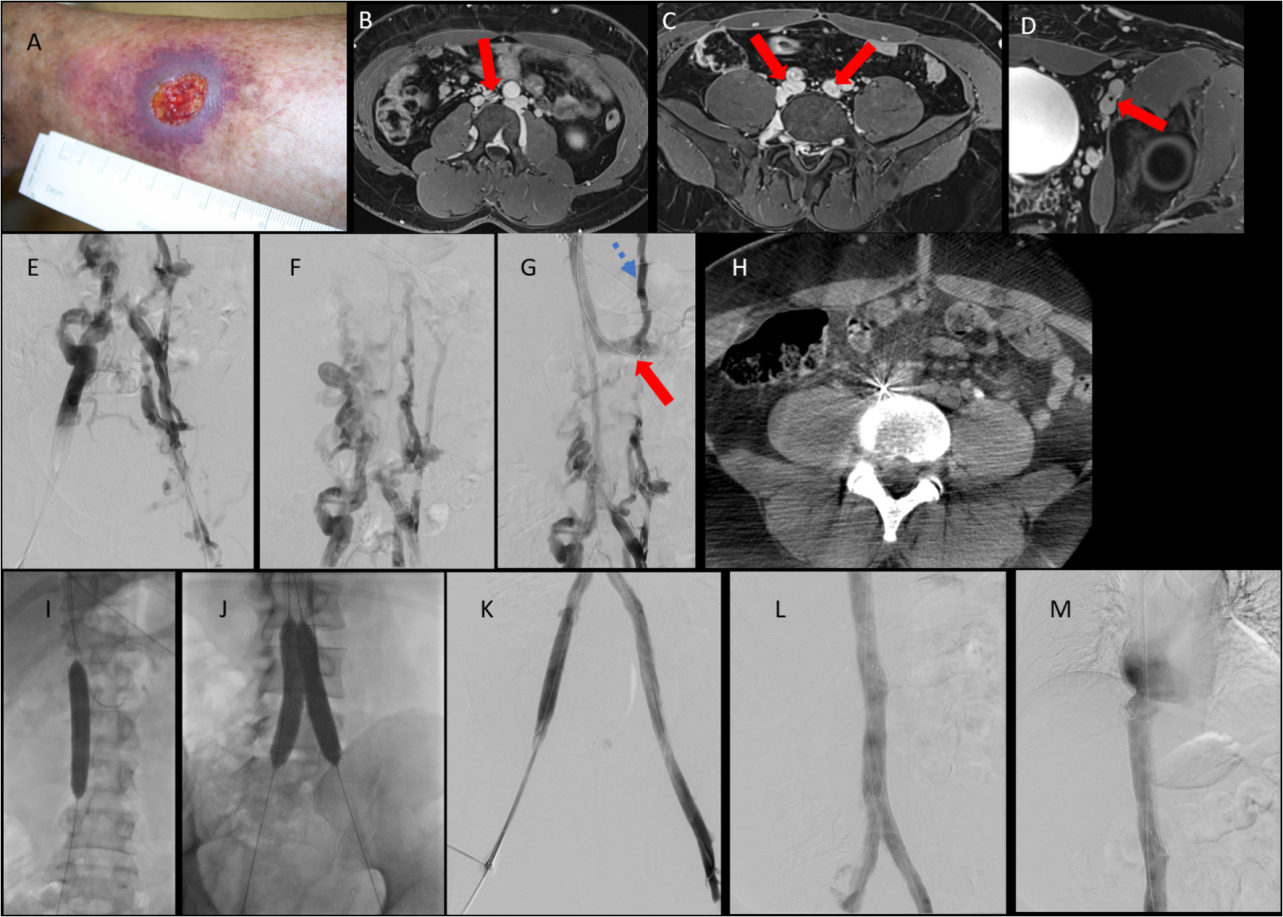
40-year-old male patient with severe post-thrombotic syndrome and ligation of the inferior vena cava. A: Clinical image of the left leg, with swelling and venous ulcer. B: MR venography with IVC remnant (red arrow) and venous lakes C and D: iliac veins with post-thrombotic synechiae down to the level of the left external iliac vein. E and F: Corresponding venography images of the diseased caval confluence (E) and lumbar collaterals (F). G: Wire passage of the occlusion after access via right and left femoral vein and right IJV. Catheter in the left renal vein (red arrow) with partial venous drainage of the left kidney via collaterals (blue dotted arrow). H: Cone-beam CT confirming wire path in the IVC remnant. I: ballooning of the occlusion to prepare stent implantation. J: Reconstruction of the IVC confluence using two parallel 14 mm self-expanding Venovo Stents (BD Bard) in a large 24mm Sinus XL Stent. Hypoplastic retrohepatic IVC was stented using a 28 mm Sinus XL Stent (not shown) to ensure outflow. K, L and M: Final venogram after reconstruction of the iliac vein (K), iliocaval confluence and distal IVC (L) and retrohepatic IVC (M)
